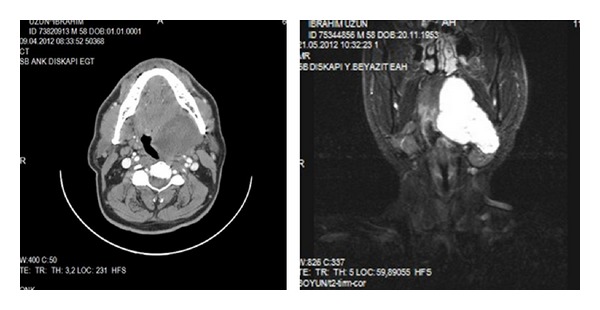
Preoperative CT scan and MRI demonstrate a hyperintense mass.
